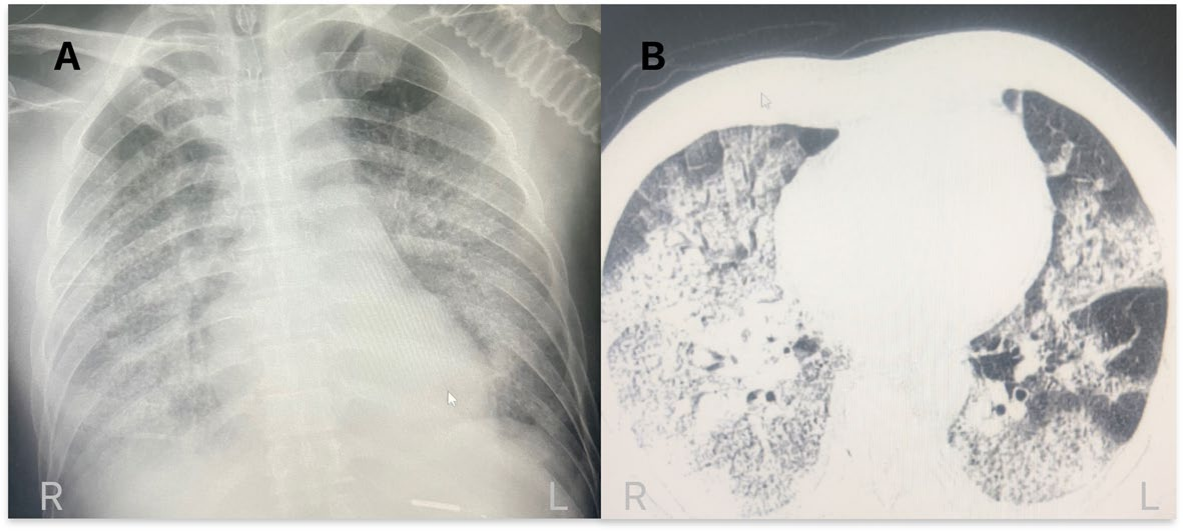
Chest CT in this case of a 51-year-old man with H10N3: Multiple patches and flaky shadows in both lungs, slight thickening of the pleura bilaterally, and a small amount of pleural effusion
